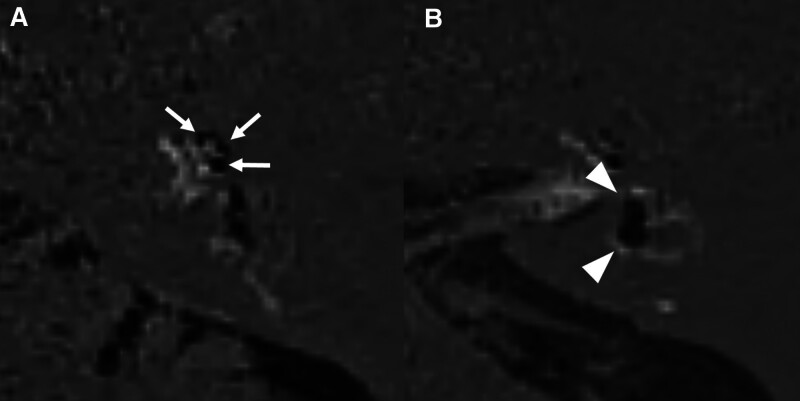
Inner ear magnetic resonance imaging (MRI) of a 60-year-old male with dizziness and left tinnitus. (A) Grade II cochlear hydrops is detected on a 0.1 mm axial hybrid of reversed image of positive endolymph signal and native image of positive perilymph signal (HYDROPS) image of inner ear MRI at cochlear level, in which a nodular black cutout of the scala vestibuli (arrows) is visible. (B) Grade II vestibular hydrops is detected on a 0.1 mm axial HYDROPS image at vestibular level, in which a complete confluence of saccule and utricle (arrowheads), occupying almost all the vestibule, is visible, but with visible enhancement of the perilymphatic rim.
